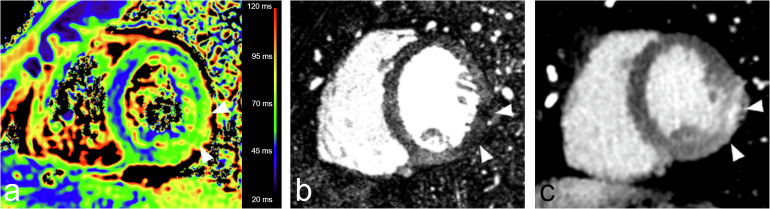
A 23-year-old man presenting to the emergency department with chest pain, a troponin level peak at 16,772 ng/mL, and a low risk of coronary artery disease. CT was performed on arrival, and magnetic resonance imaging was performed the following day. Increased values of T2 in the inferolateral wall of the left ventricle (a) corresponded to a HypoArt–HyperLE lesion: hypodensity on the arterial phase (arrowheads in b) and corresponding epicardial hyperdensity (arrowheads in c) on the late phase on monoenergetic images at 40 keV. The final diagnosis was of viral AM. HypoArt–HyperLE, Hypodense at arterial phase and hyperdense at late enhancement phase; HypoArt–Nor, Hypodense at arterial phase and isodense at late enhancement phase; NorArt–HyperLE, Isodense at arterial phase and hyperdense at late enhancement phase
